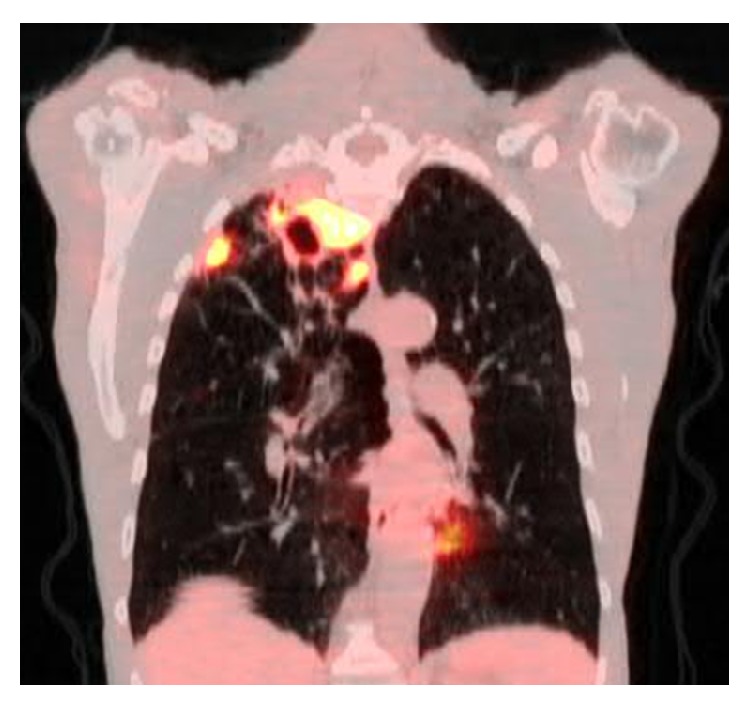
The ^18^F-FDG PET scan demonstrated hypermetabolic areas at the peripheral consolidation (SUV 7.4) and the apical cavitary mass (SUV 12.8) of the right upper lobe.
